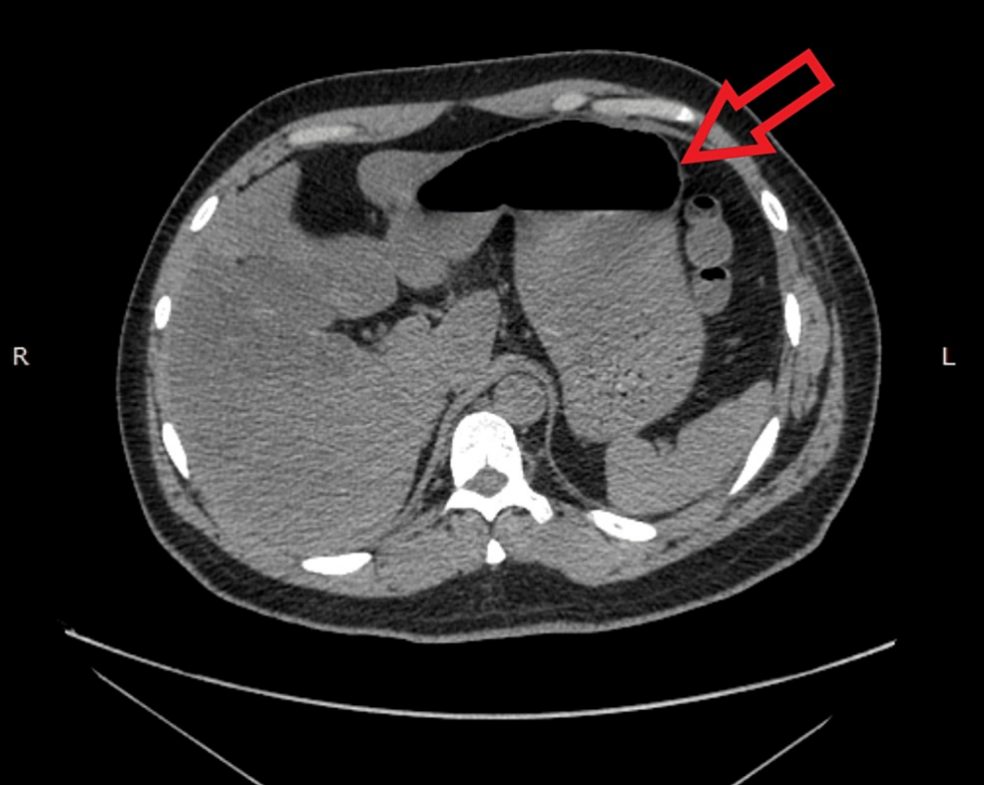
Computed tomography scan done 20 days after the surgery (axial view) showing the stomach (red arrow) in the abdominal cavity, and no evidence of diaphragmatic herniation
